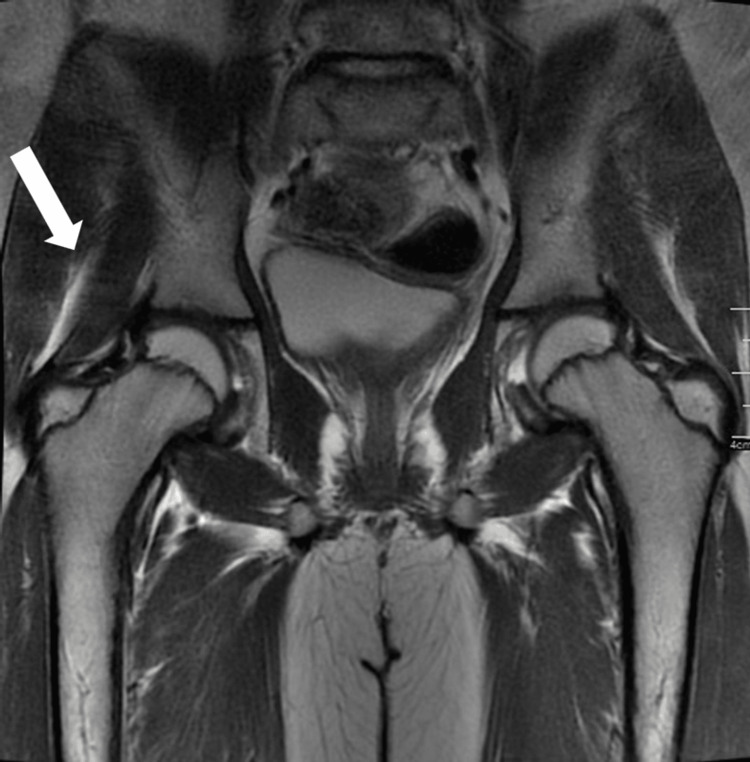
MRI of the pelvis showing normal soft tissues (white arrow)
